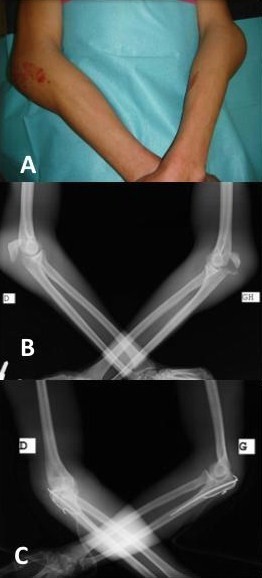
A) deformation of two elbows; B) Radiography objectified a fracture of the both olecranon; C) surgical treatment by Tension-band wiring fixation
